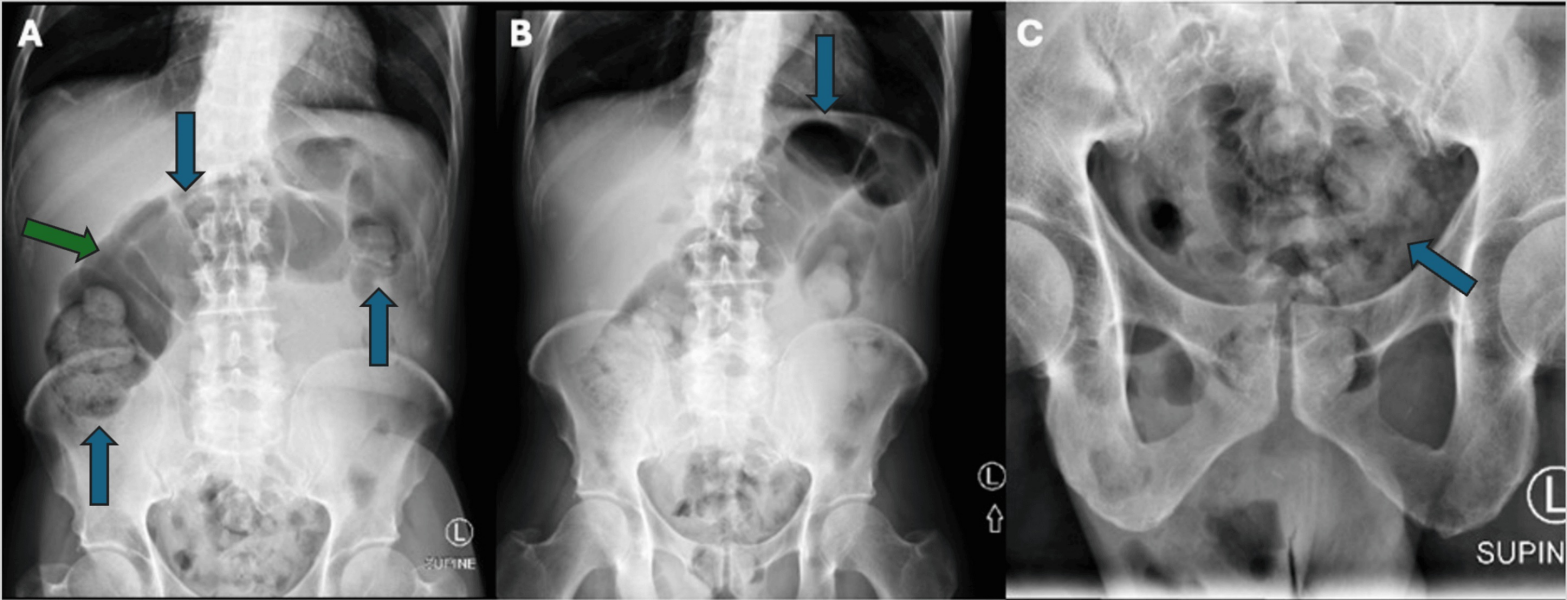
Abdominal X-ray The S2 (A), S4 (B), and S5 (C) views of an abdominal X-ray demonstrate a large volume of stool, especially in the rectum; splenic flexure; and hepatic flexure, respectively. Transverse colon distension is noted with bowel gas (green arrow) measuring 9 cm without evidence of free air.
